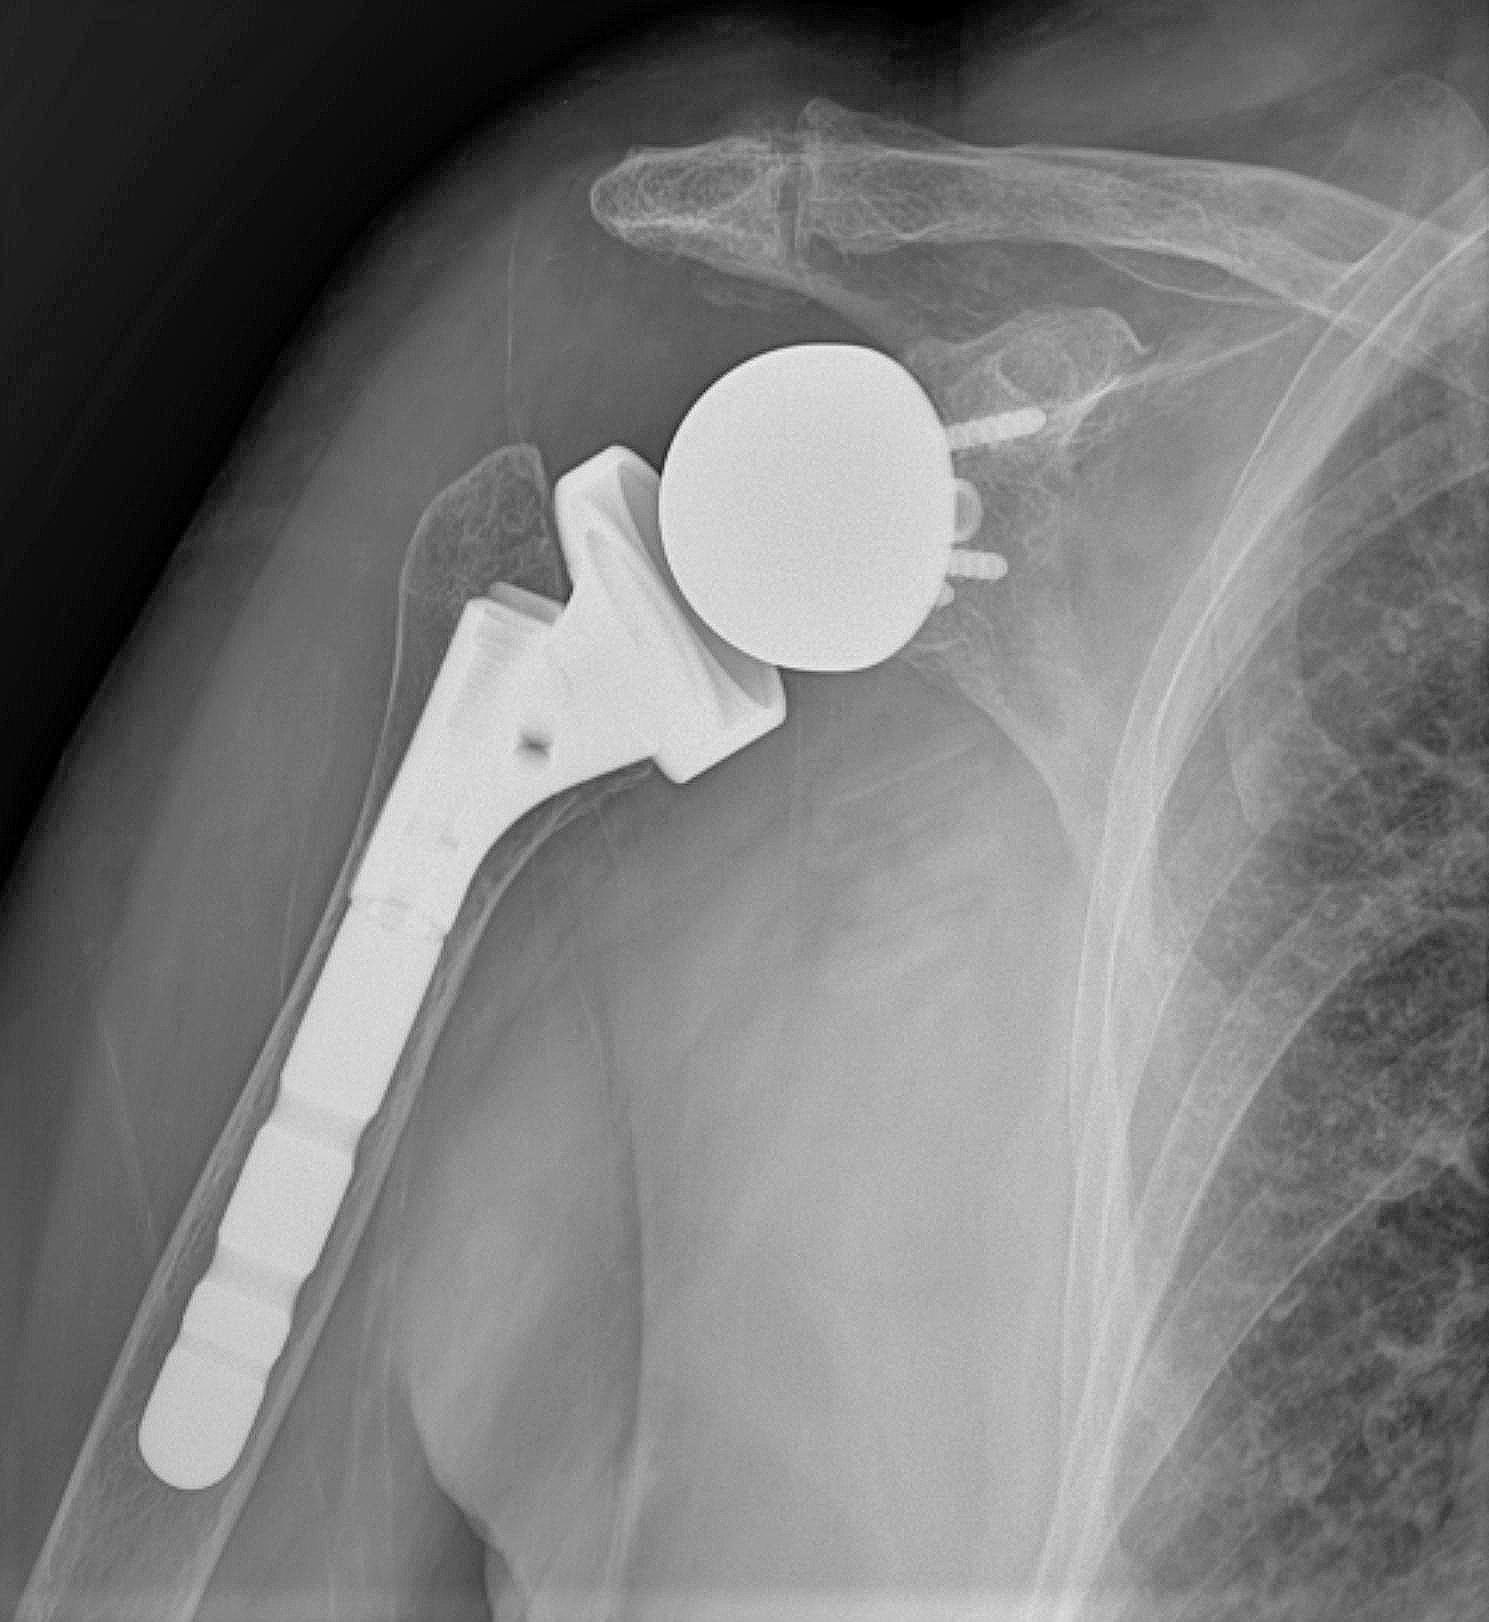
1st-year post-operative x-ray
